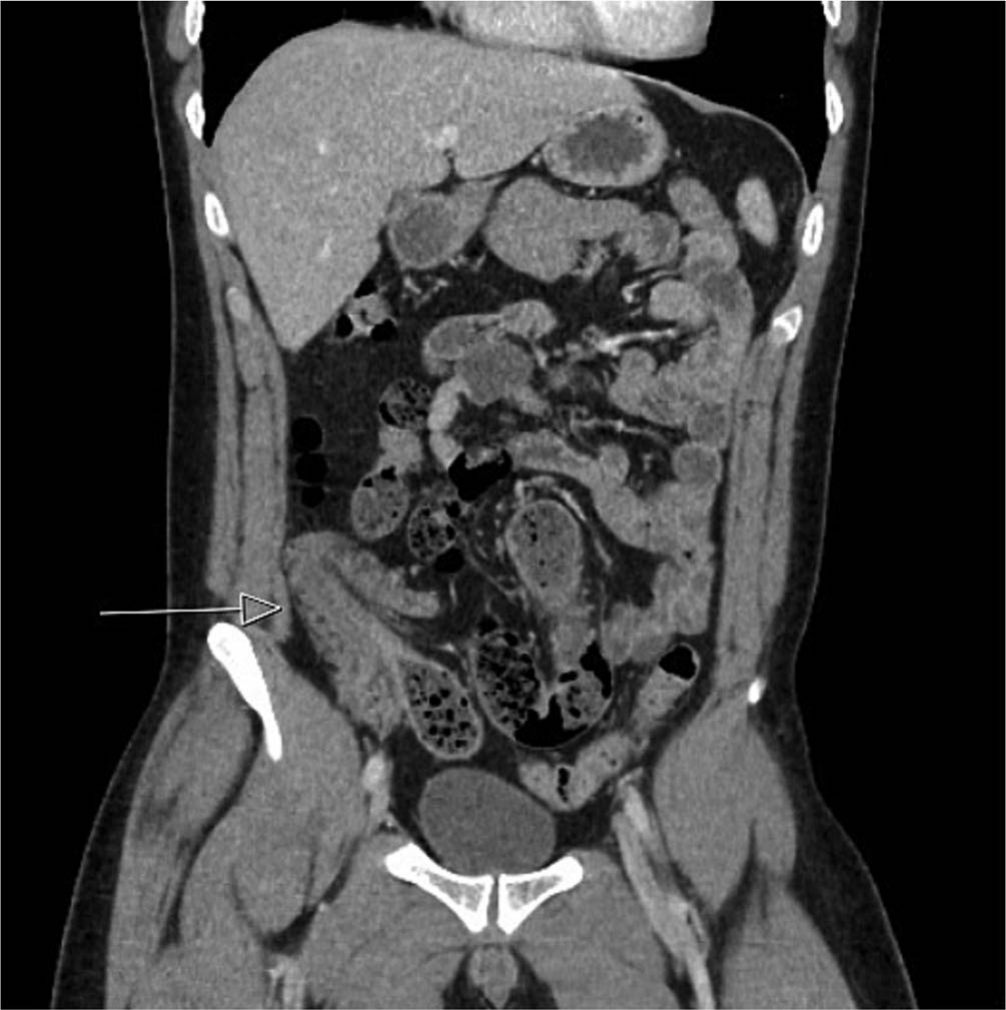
Initial CT imaging, axial cut, showed an abnormal ileocolic configuration with intussusception of the distal ileal loop through the cecum.
